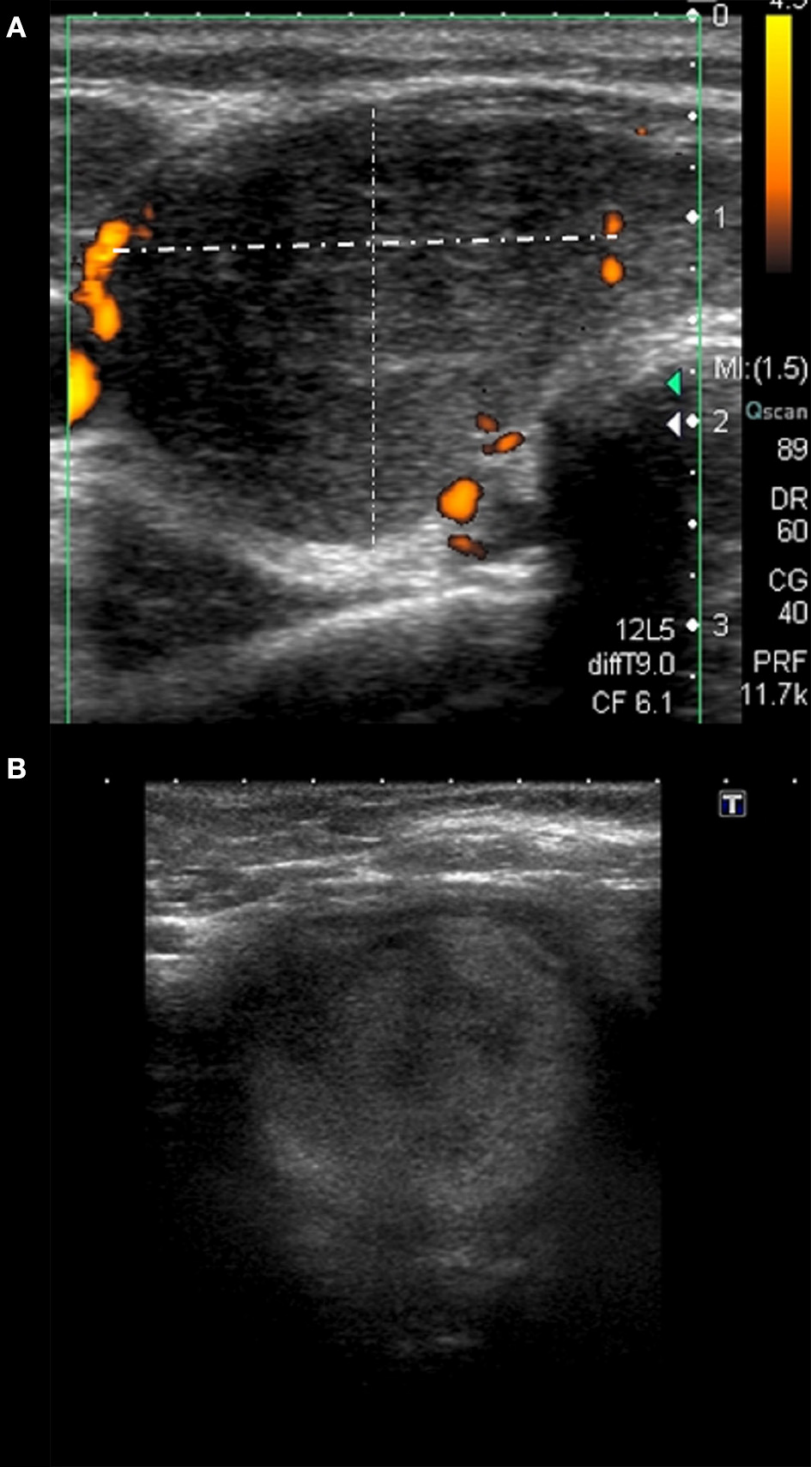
Different sonographic pattern observed in patient with HLA-B*18:01 only. (A) Homogeneously hypoechoic single area filling the whole right lobe; (B) moderately hypoechoic single SAT area filling the whole thyroid lobe, mimicking a nodule affecting the whole lobe.
